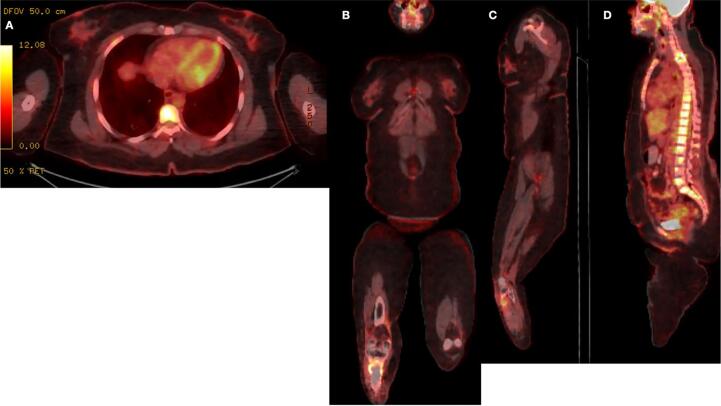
PET-CT scan (A, B, and C) showing no metastasis. Metabolic regression of the hypermetabolic bony lesion involving L-3 lumbar vertebra (D), stable diffuse mild activity in the right upper tibia along the bone graft with metallic fixation suggesting post-operative/reactive inflammatory changes (B, and C).
